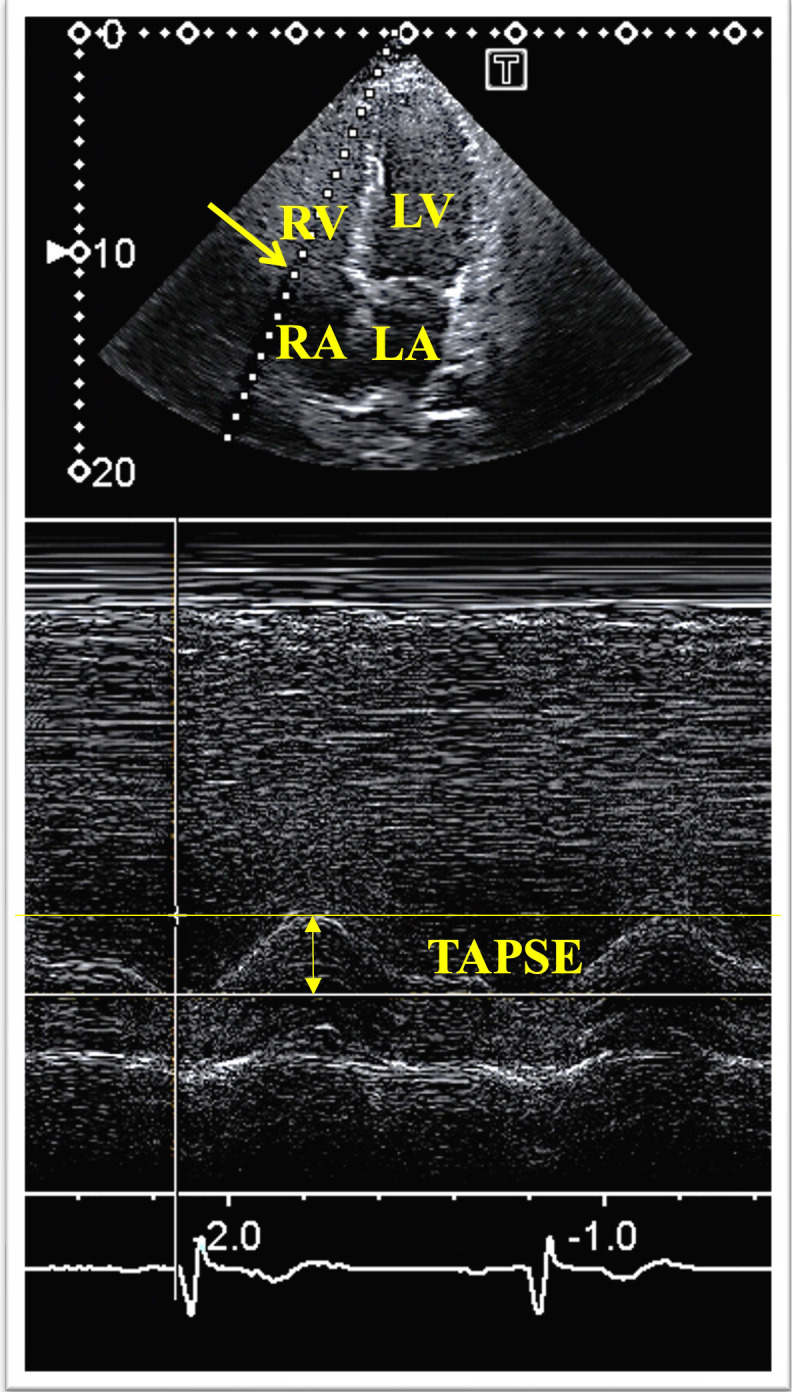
M-mode echocardiography-derived measurement of tricuspid annular
